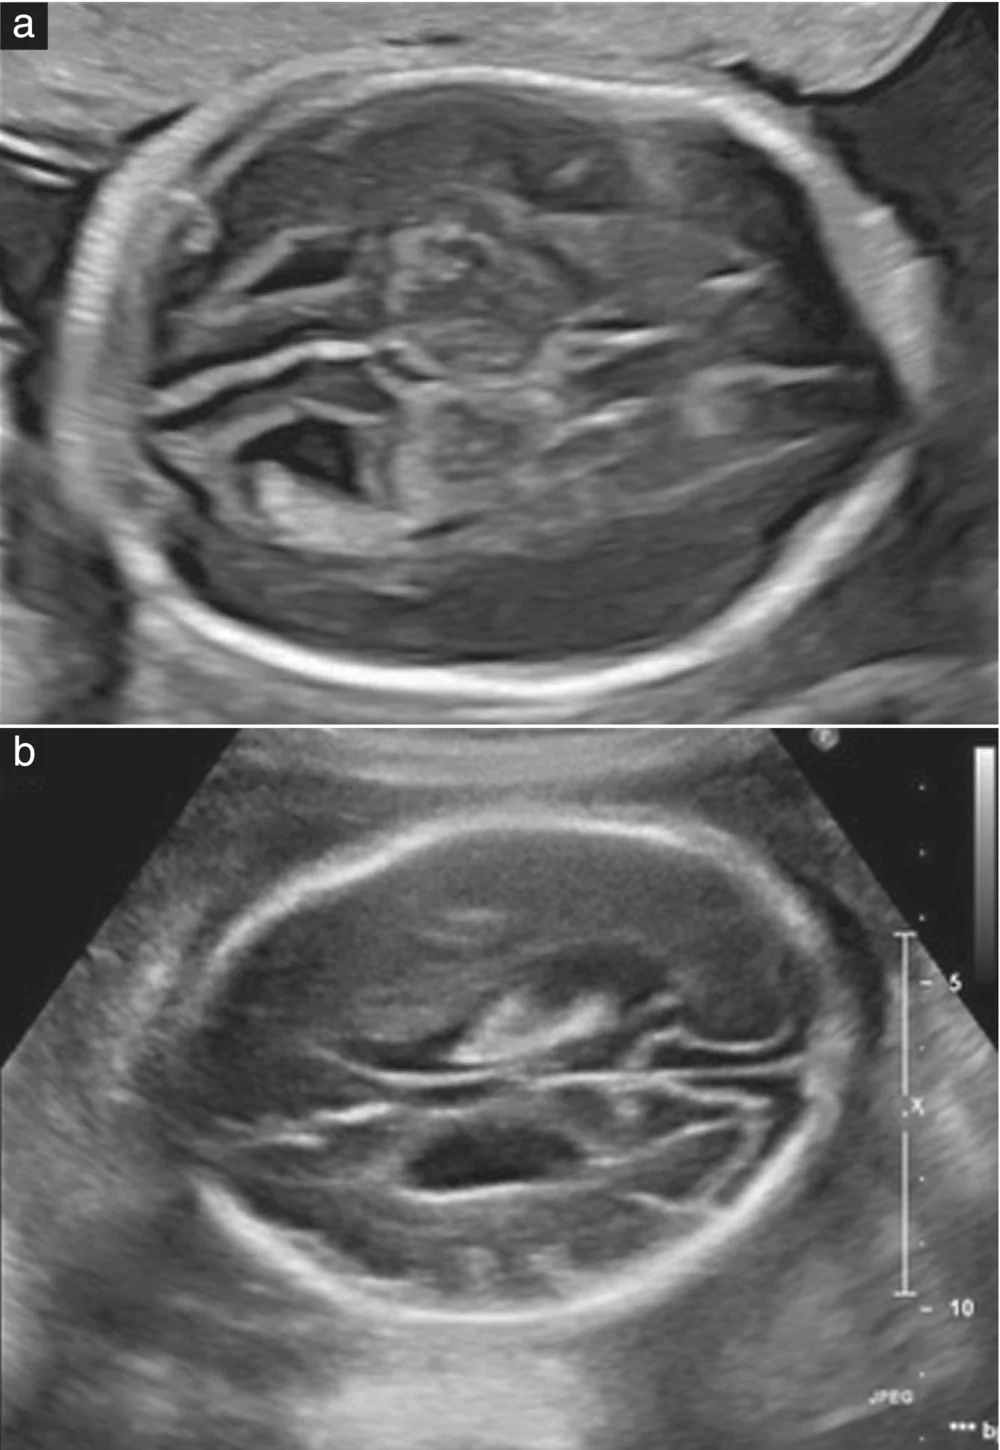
Transabdominal ultrasound imaging of fetus at 24 + 0 weeks' gestation in axial view, demonstrating distortion of the posterior interhemispheric fissure (IHF) prior to fetal myelomeningocele repair (a) and the same fetus at 31 + 1 weeks' gestation in axial view, demonstrating a normal‐appearing IHF following fetal myelomeningocele repair (b).
